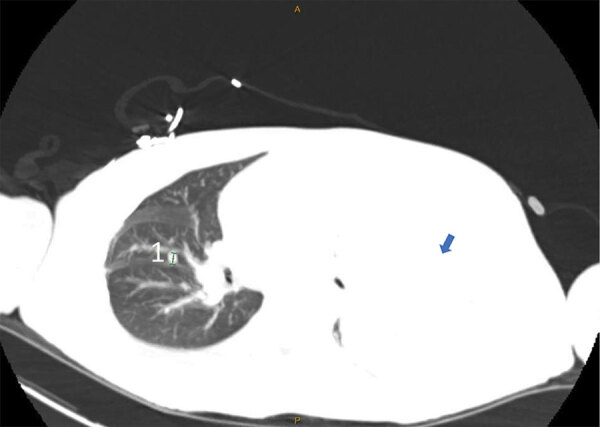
Computed tomography scan of lung showing right-sided pulmonary nodule (marked as 1) and large left pleural effusion (blue arrow) in patient with severe mpox and untreated HIV, Baltimore, Maryland, USA.
